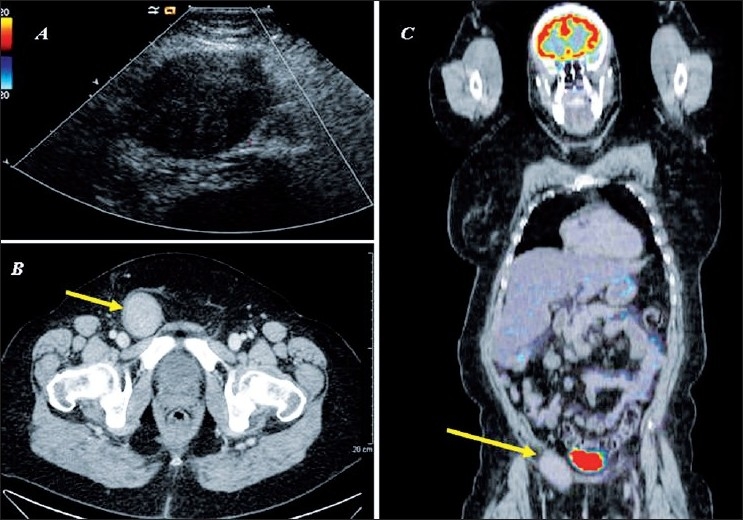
(A) Ultrasound showing a 6.3 × 4.2 cm hypoechogenic, nonvascularised inguinal tumour. (B) CT scan showing a 4 × 6 cm, hyperdense vascularised tumour. (C) PET-CT scan: there is no uptake of labeled glucose in the inguinal canal
